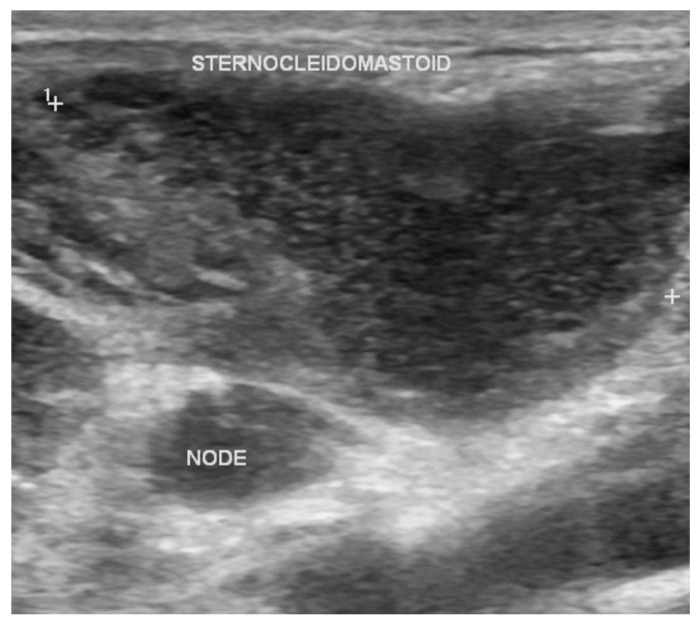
Ultrasound image of the neck demonstrating an enlarged hypoechoic cervical lymph node adjacent to the sternocleidomastoid muscle, consistent with cervical tuberculous lymphadenitis. Case courtesy of Maulik S Patel, Radiopaedia.org, rID: 19137, licensed under CC-NC-BY-SA 3.0 [50].
